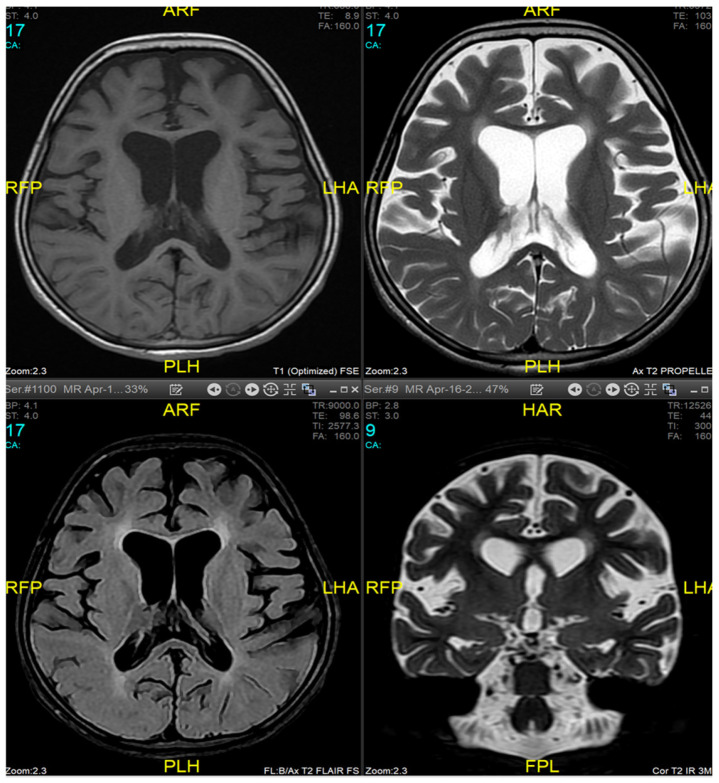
Brain MRI at 9 years of age (16 April 2024). Generalized atrophic changes of the brain, characterized by supra and infratentorial ventricular dilatation, prominent cortical sulci, and extra-axial CSF spaces such as Sylvian fissures and basal cisterns. The diffuse deep periventricular white matter showed high signal intensities on T2/FLAIR (fluid-attenuated inversion recovery) sequences. There was global thinning of the corpus callosum.
